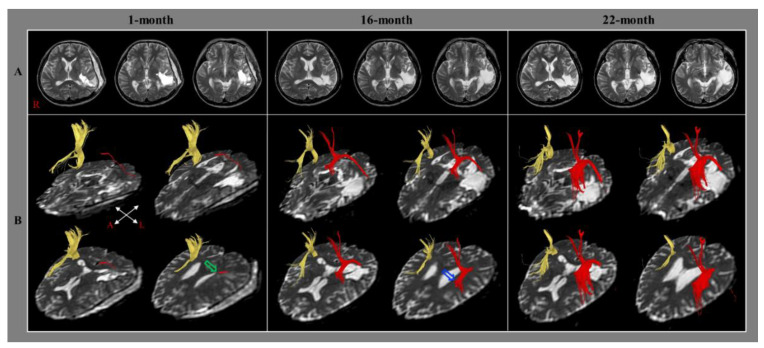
(A) Brain MR images at 1, 16, and 22 months after onset show leukomalatic lesions in the left parieto-temporal areas (R: right). (B) DTT for the arcuate fasciculus (right AF, yellow; left AF, red). The right AF passes through the known pathway of the AF. On 1-month DTT, the left AF showed discontinuation (green arrow) after originating from the Wernicke area. In contrast, on 16-month DTT, the injured left AF was thickened and elongated to around the left Broca area; however, discontinuation (blue arrow) of the left AF was observed around the left Broca area without termination at the left frontal area. On 22-month DTT, the discontinuation of the left AF was elongated to the left Broca area and the left AF was thickened (reprinted with permission from Ref. [21]).
